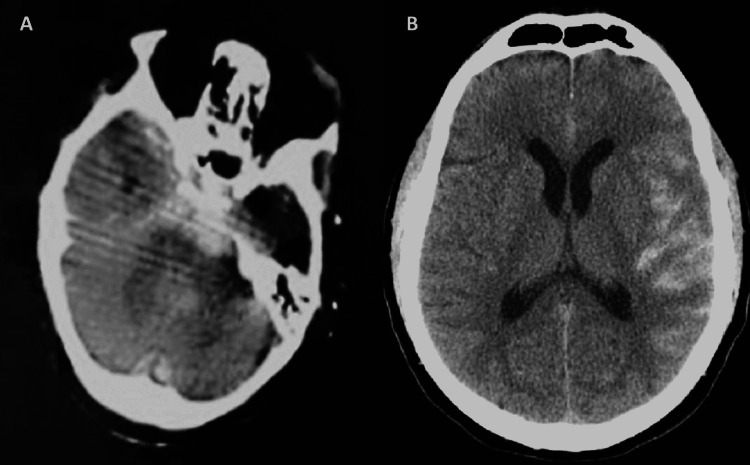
(A) Axial orbital CT scan showed left lateral orbital wall dislocation to the posterior-lateral side. (B) Axial brain CT scan revealed a left-sided subarachnoid hemorrhage CT, computed tomography.
